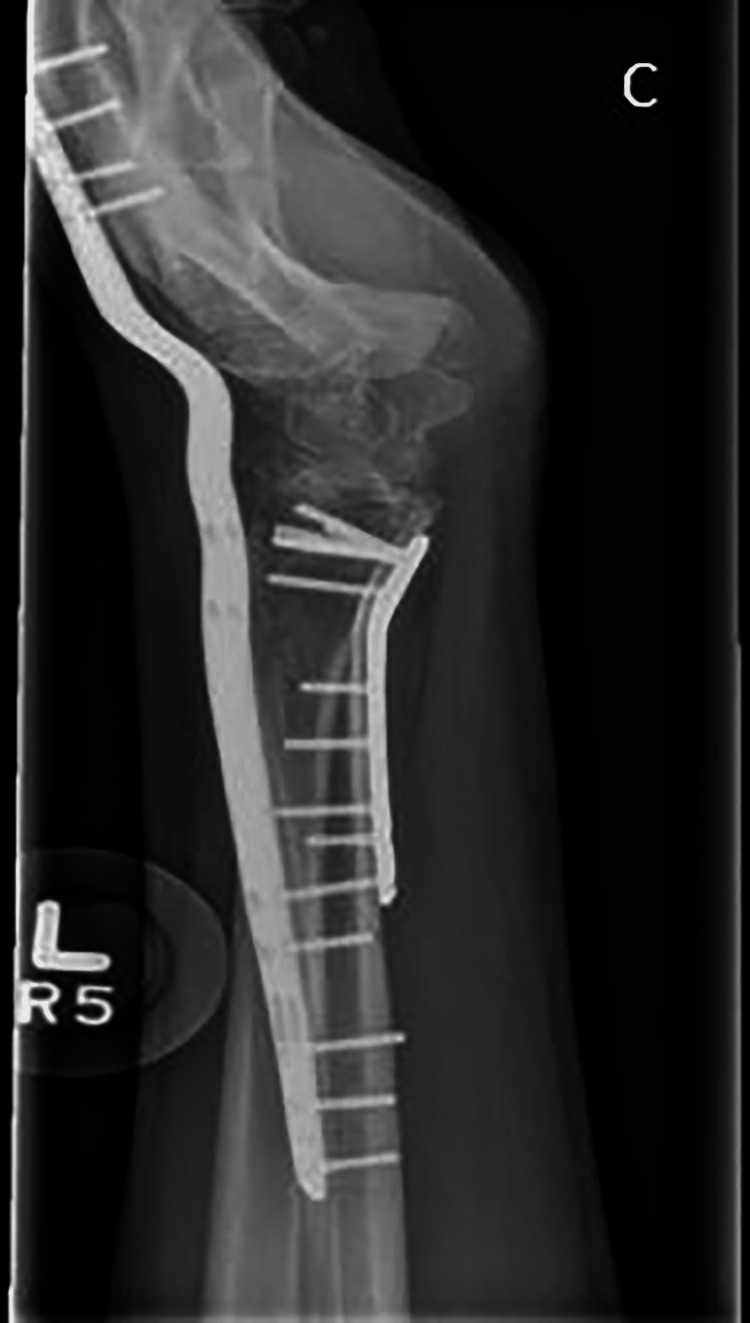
Lateral radiograph after the first stage of surgery. Length alignment and rotation has been resorted through the volar plate and dorsal fixator.
